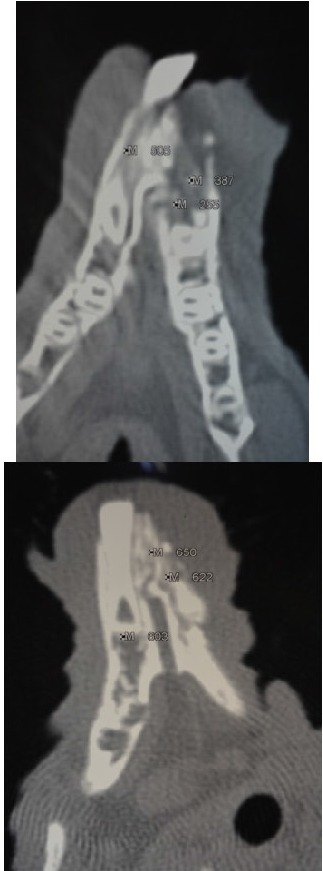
CT investigations at 1 month and 3 months after surgery showing central and peripheral bone density of mandible
